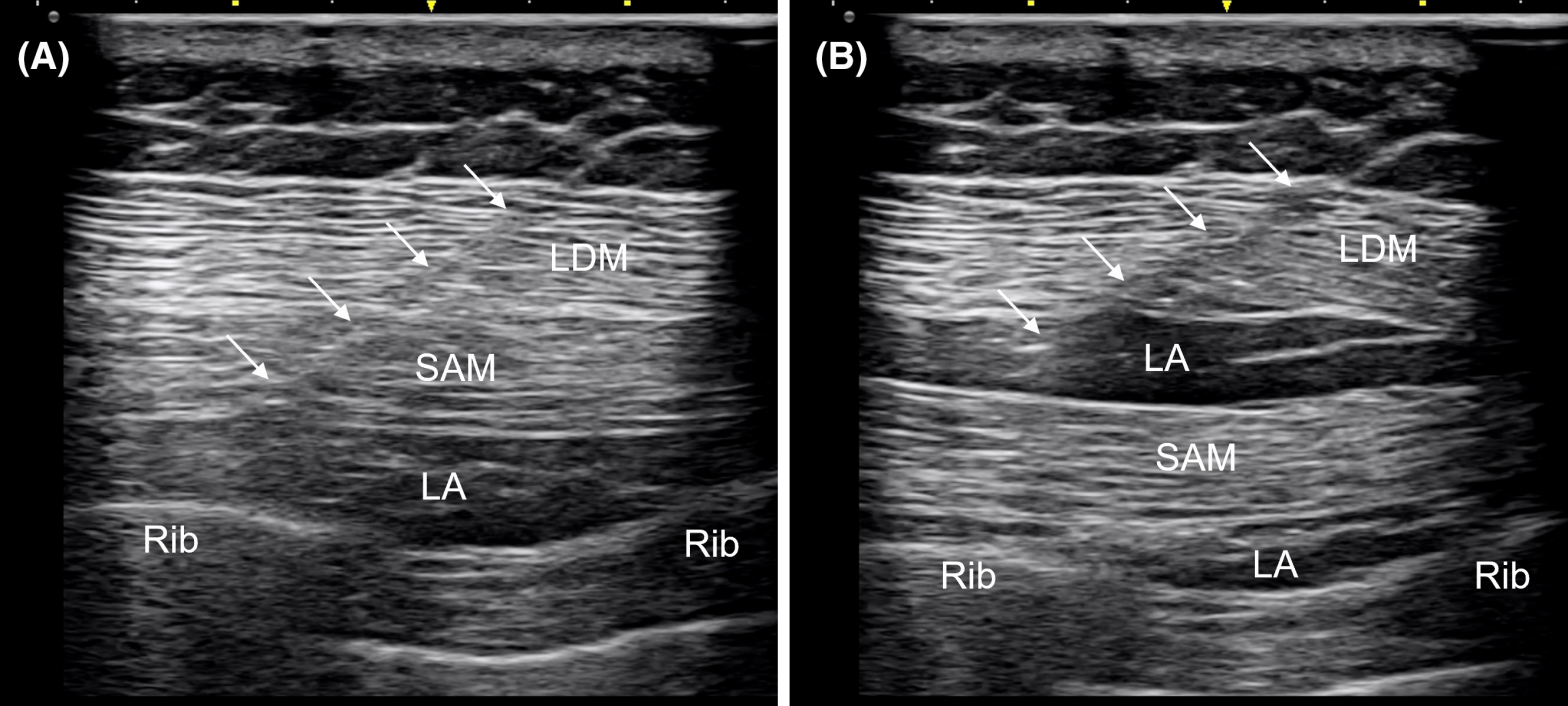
Ultrasound images of the serratus plane block. Local anesthesia is injected into the deep plane (A) and superficial plane (B) through a Tuohy needle (arrows). LA, local anesthetics, LDM, latissimus dorsi muscle, SAM, serratus anterior muscle.
